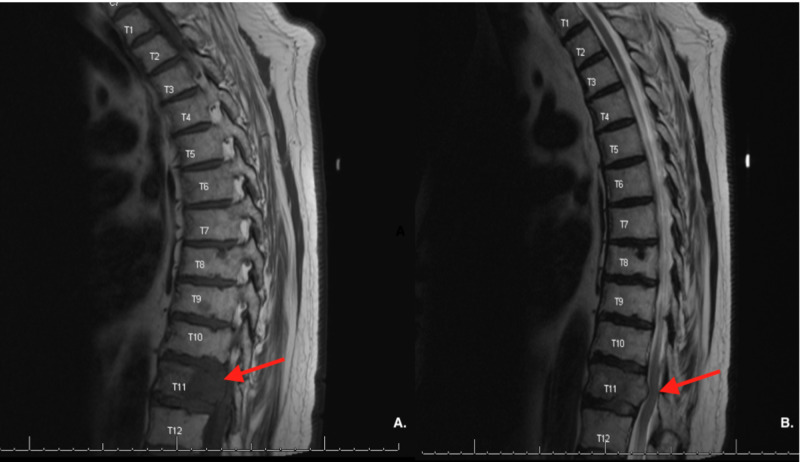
A: MRI of the lumbar spine revealing lytic lesions at T11. B: moderate compression of thoracic canal and spinal cord by mass.
